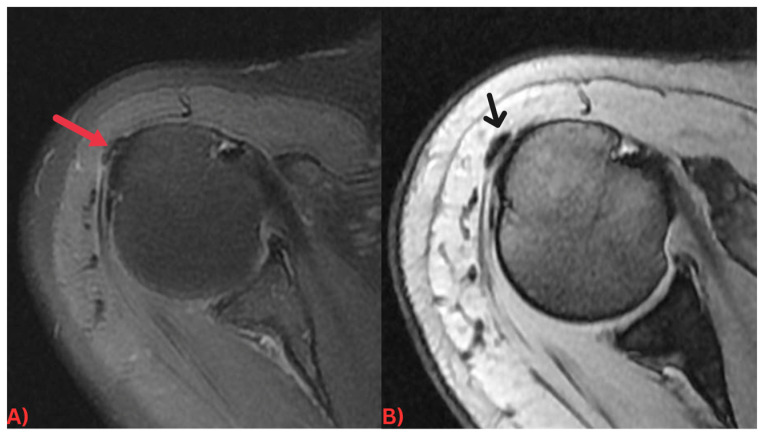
(A) Axial PD fat-saturated MRI image and (B) axial GRE sequence MRI of the right shoulder demonstrating a small well-defined low signal intensity calcification at the insertion of the supraspinatus tendon (red arrow). Note how the calcification is more visible and conspicuous on the GRE sequence (black arrow) due to a blooming artifact from the calcification.
